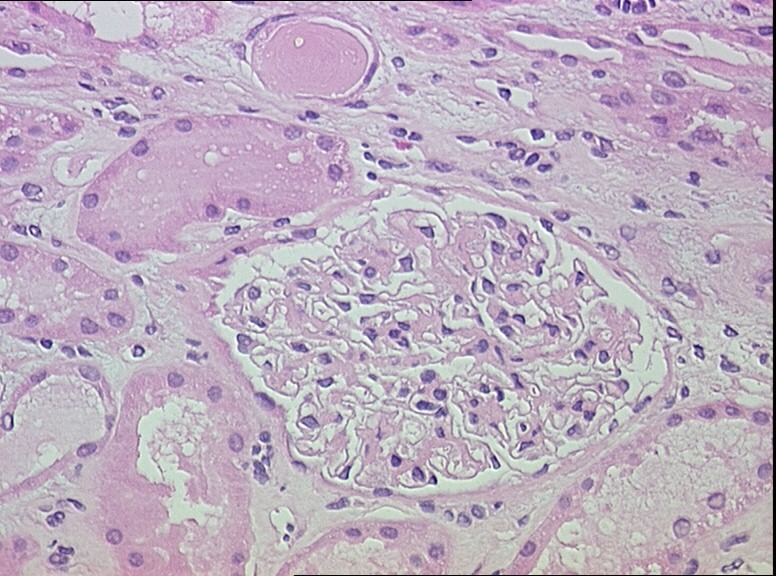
One week later hemodialysis was started due to further deterioration of kidney function (creatinine 6.7 mg/dL). Laboratory and renal sonographic follow-up were performed for five months and dialysis was suspended when the patient experienced partial recovery of renal function (creatinine 4.1 mg/dL, GFR 16 mL/min). Ultrasound images showed less enlarged kidneys, but also a progressive thinning of renal cortex and a reduction in cortico-medullary differentiation. RIs remained elevated. The patient’s creatinine level remained elevated, but stable.
